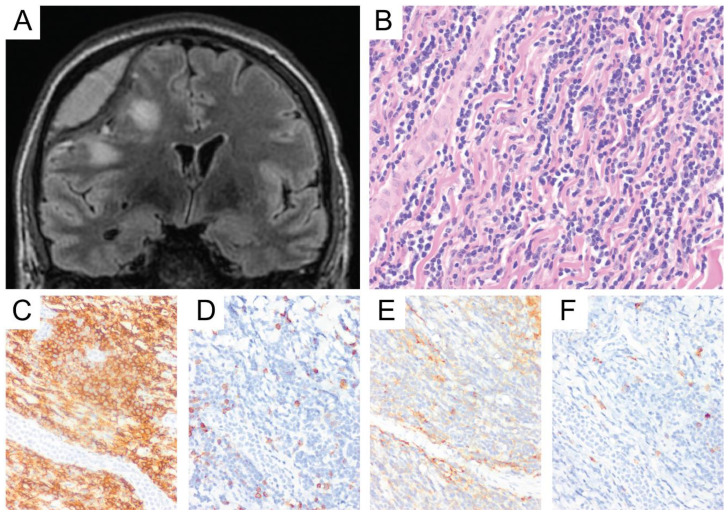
MALT lymphoma of the dura. This lymphoma typically presents as an enhancing dural-based mass (so-called “dural tail”), often mistaken for meningioma (A, coronal section, magnetic resonance imaging FLAIR sequence). The tumor cells are small-to-medium-sized with irregular nuclei and abundant cytoplasm and are embedded within a background of fibrous dura (B, original magnification ×400). The tumor cells are often positive for CD20 (C) and negative for CD5 (D), CD10 (E), and CD23 (F) (B–F, original magnification ×200).
